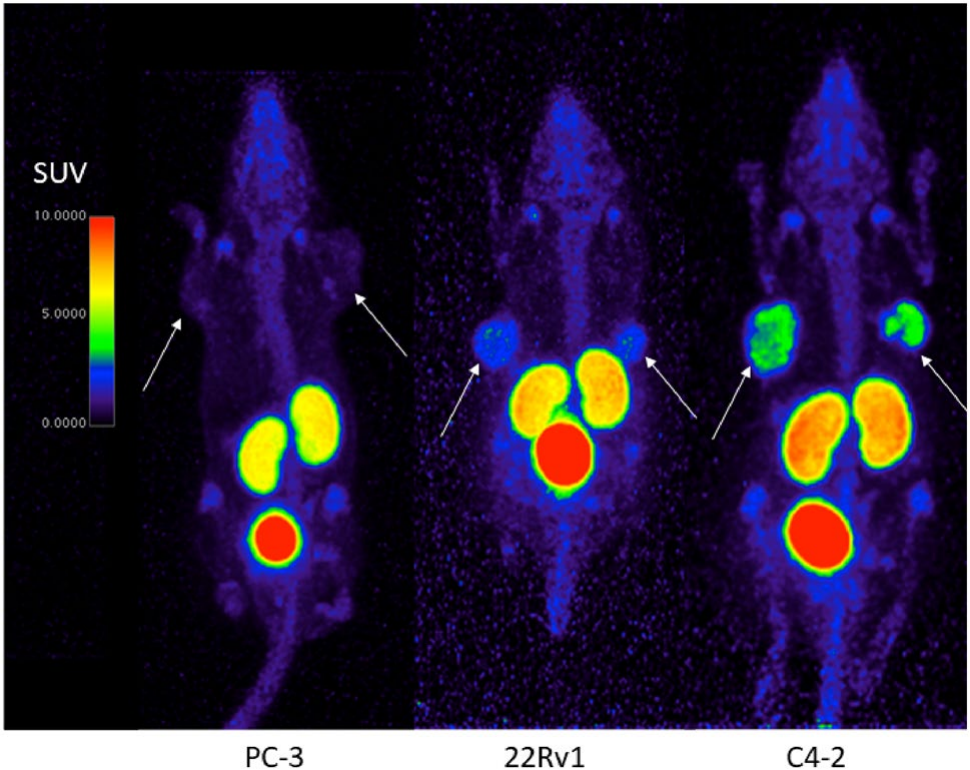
Representative PET images of PC-3 (left), s22Rv1 (middle) and C4-2 (right) xenografts 60 min p.i. of [^18^F]AlF-PSMA-11 with medium MA_app_. Arrows indicate tumors. There was no activity uptake detectable in PC-3 tumors, C4-2 and 22Rv1 tumors were visible, although the latter showed less tumor contrast. Color maps were generated using Horos v4.0.0, https://horosproject.org/.
